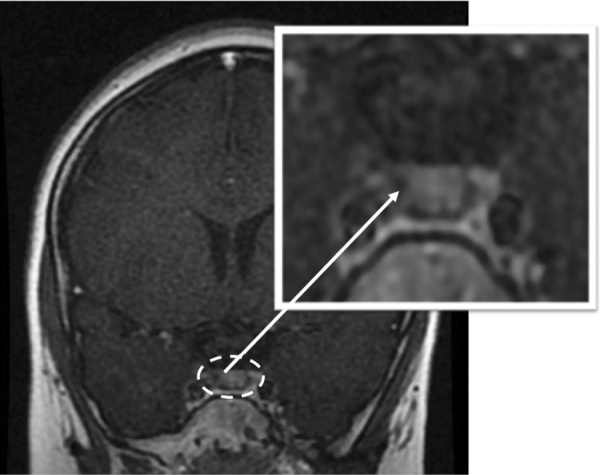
Рисунок 2. МРТ головного мозга в 6 лет 9 месяцев. Пунктирной линией выделен аденогипофиз, стрелкой указано локальное снижение контрастирования в правой части аденогипофиза, убедительных данных за наличие аденомы гипофиза нет.Figure 2. Brain MRI at  6 years 9 months. The dotted line indicates the adenohypophysis, the arrow indicates a local decrease in contrast in the right side of the adenohypophysis, there is no convincing evidence for the presence of pituitary adenoma.
